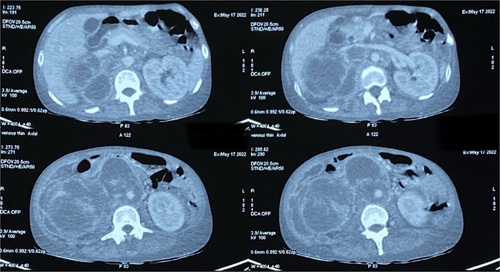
CECT report showing right renal mass. CECT, contrast enhanced computed tomography
